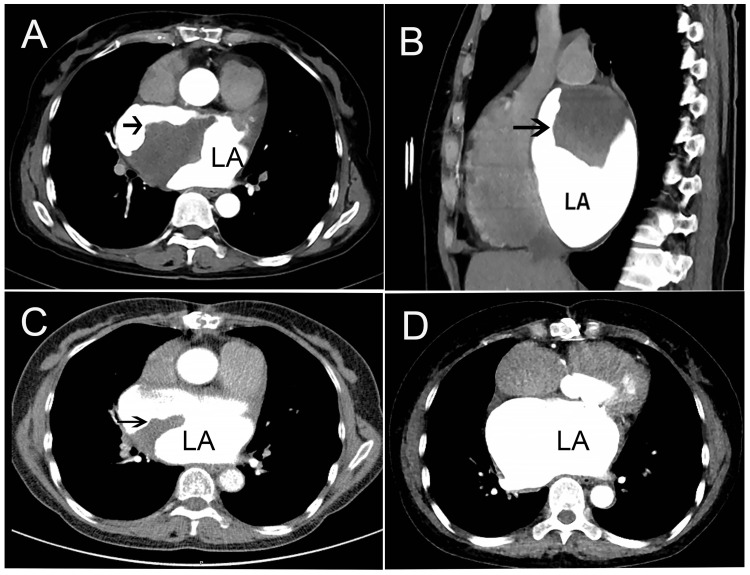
Cardiac CT demonstrating the resolution of a left atrial (LA) thrombus. Transverse (A) and sagittal (B) views showing a thrombus within the LA (arrows), with an irregular contour, measuring 79 × 55 × 45 mm. (C) Follow-up CT at 3 weeks demonstrated a marked reduction in thrombus size (arrow). (D) Follow-up CT at 3 months confirmed complete resolution of the thrombus. CT: computed tomography.
